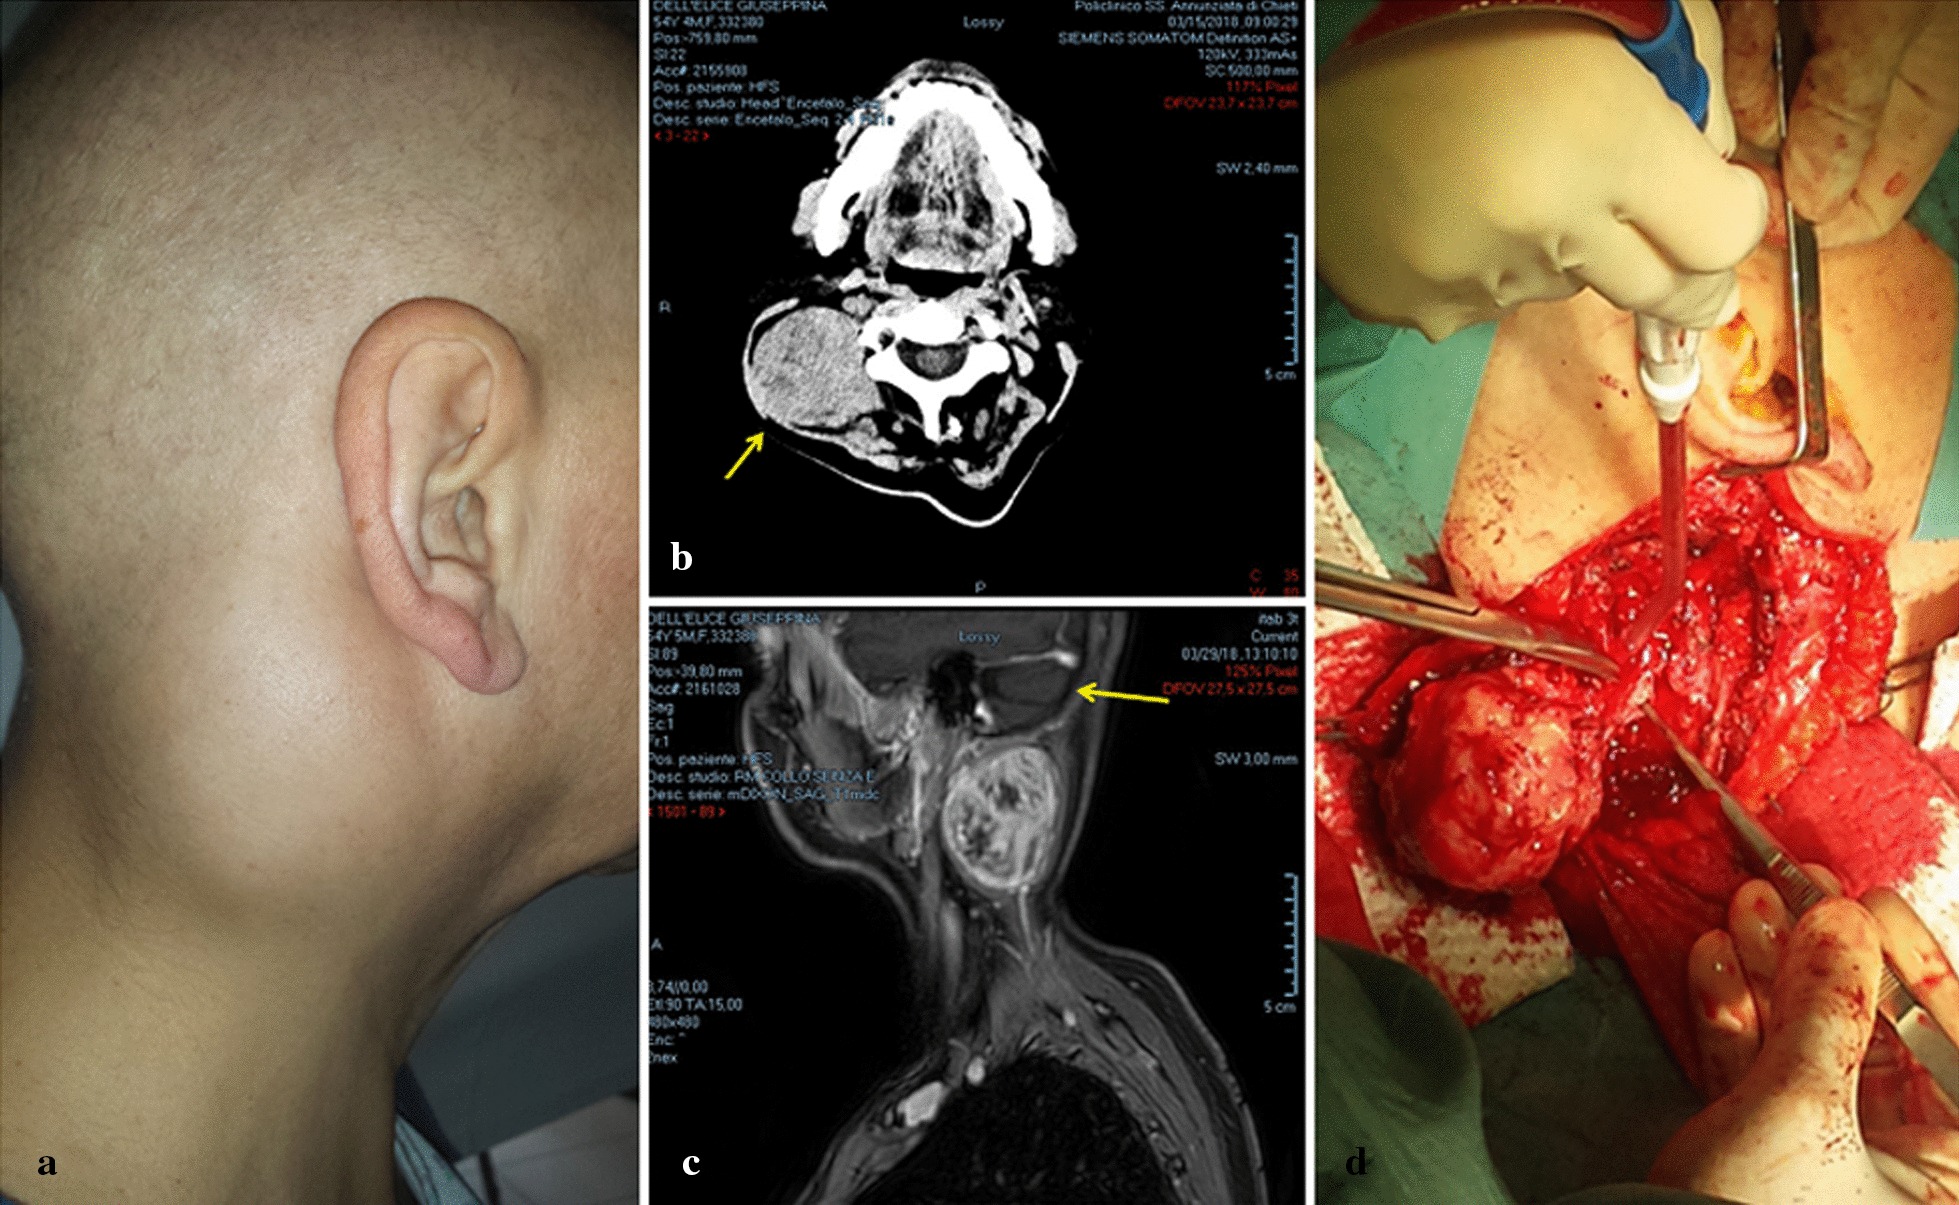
Representative preoperative, CT scan, MRI, and surgical excision images. a Preoperative photograph of the mass in the right posterior neck region. b CT scan showing a solid mass (indicated by the arrow) in the posterior neck region near C1-C2. c MRI image showing a hyper-intense mass displacing paravertebral muscles (arrow), and a cleavage plane between the mass and the sternocleidomastoid muscle. d Surgical excision of the mass by posterior neck cervicotomy
